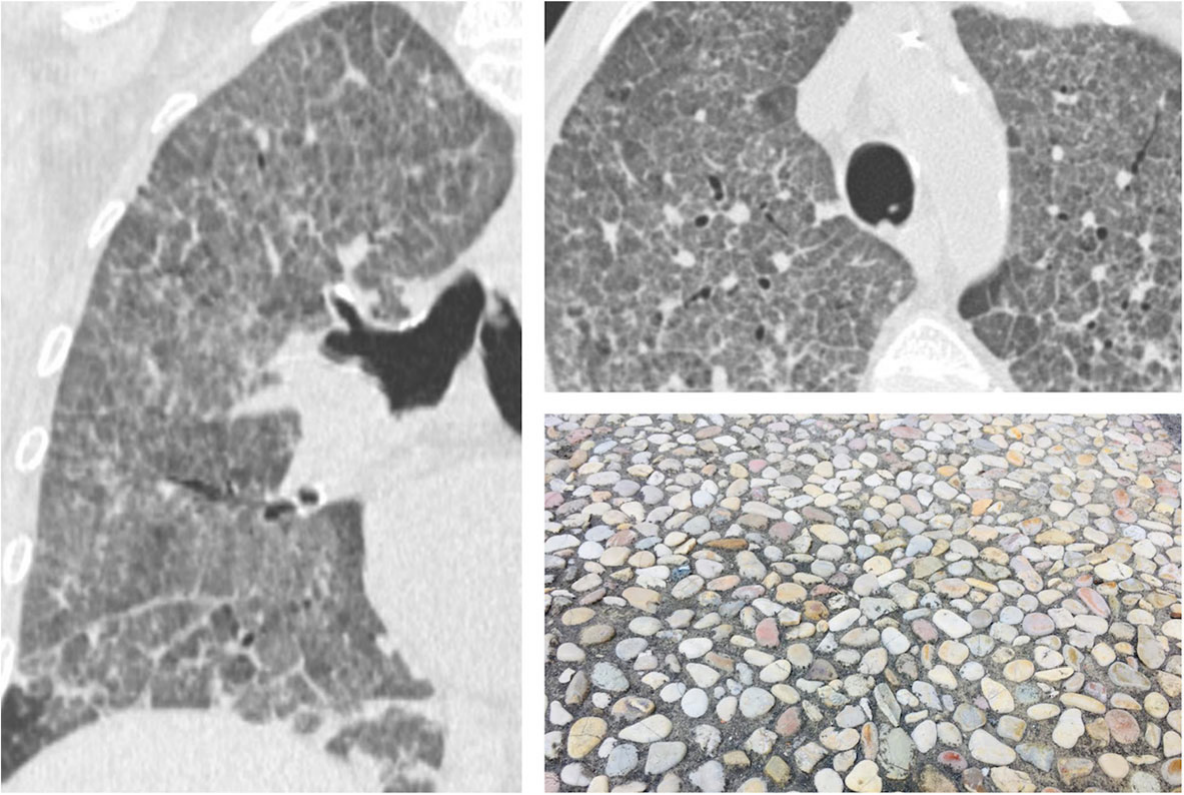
Crazy paving. Coronal (left side) and axial (right side) CT images show ground glass attenuation of the lungs—with superimposed reticulations, in a patient with *Pneumocystis jirovecii* infection. This appearance looks like the “roman crazy paving” and may be associated also with different pulmonary diseases (alveolar proteinosis, ARDS, drug-induced pneumonitis, etc.)
